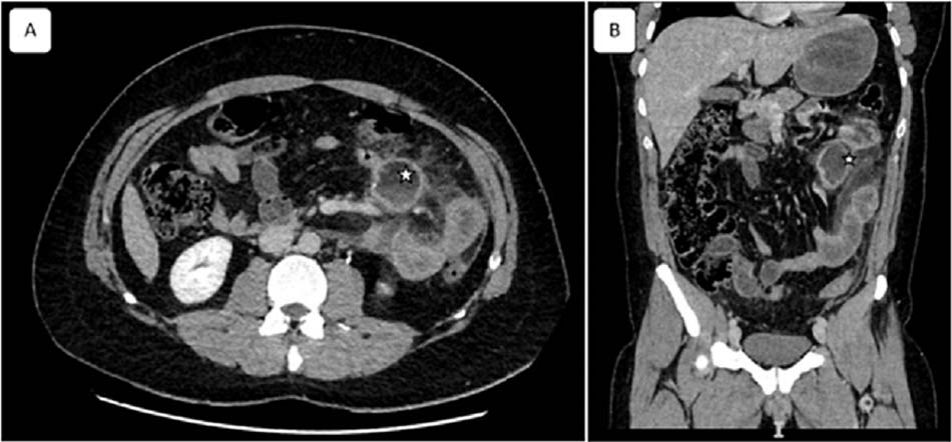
Coronal and sagittal views of computed tomography showing a mesenteric collection of 51 × 33 with mesenteric fat infiltration (asterisk) related to the peritonitis caused by the ruptured cyst. Reconstructed images: (A) axial and (B) sagittal view.
